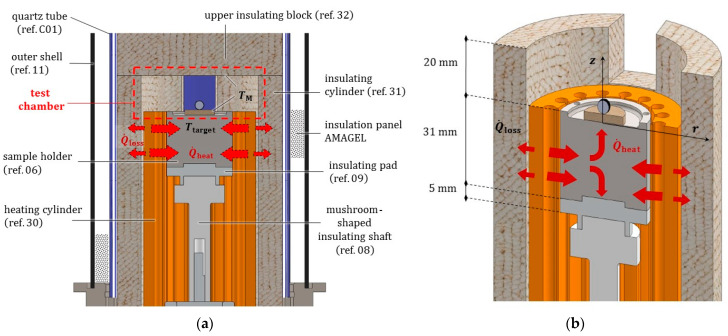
(a) Longitudinal radial section of the furnace deprived of the headers. (b) Axonometric view of the radially sectioned heating element (ref. 30), the cylindrical sample holder (ref. 06) and the insulation layer with an opening along the X-ray beam path. A pictorial representation is provided for the heat generated by the ceramic circular crown, a part of which is transferred to the sample (ref. 06) whilst another part is lost.
